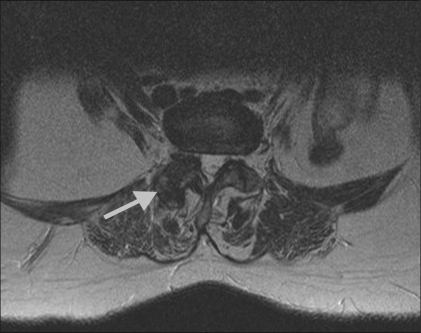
Axial T2W MRI image at L2–3 shows advanced degenerative changes in the right facet joint (arrow).
